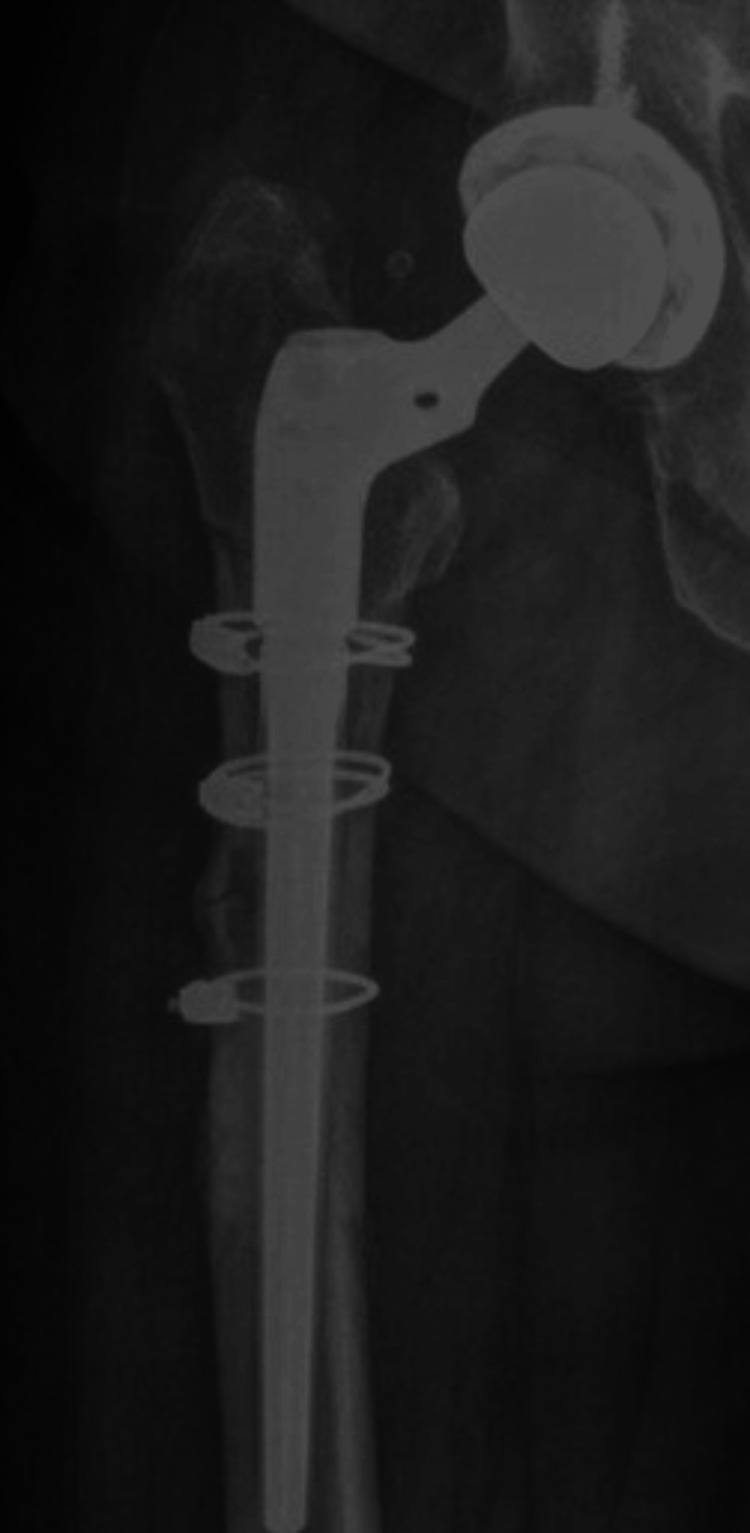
Three-month postoperative anteroposterior X-ray of the right hip. The image shows stable femoral component, osteotomy with evidence of bone healing, no evidence of loosening, and no signs of fracture or migration of the femoral stem and well-fixed implants.
